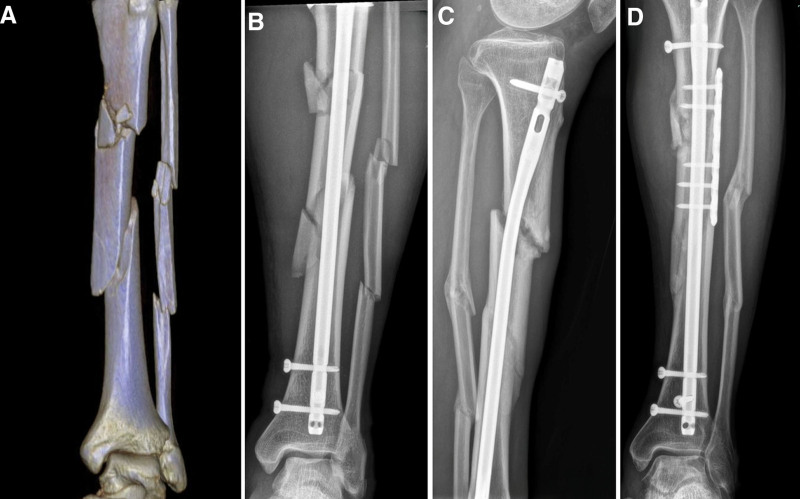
(A) A 63-year-old male patient sustained a left STF. (B) After internal fixation with the IMN, X-ray revealed good axial alignment. (C) Eight months after internal fixation with the IMN, X-ray revealed nonunion occurred in proximal fracture. (D) Two months after iliac crest bone grafting combined with small plate augment fixation, X-ray showed callus formation. IMN = intramedullary nail, STF = segmental tibia fractures.
